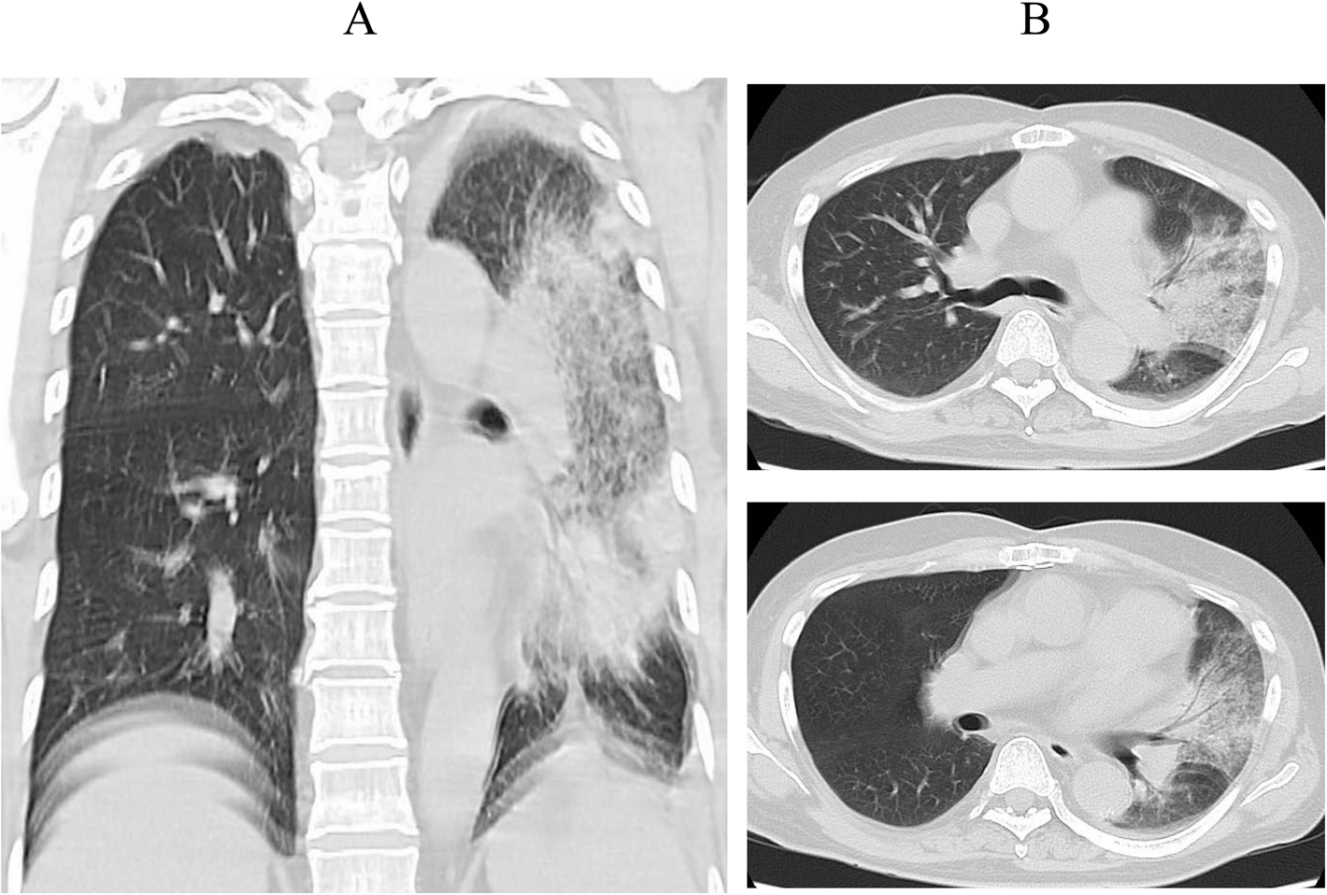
Chest computed tomography (CT) on admission. Chest CT on contrast coronal (A) and axial (B) views showing infiltrative shadow and pleural effusion in the upper and lower left lobes
